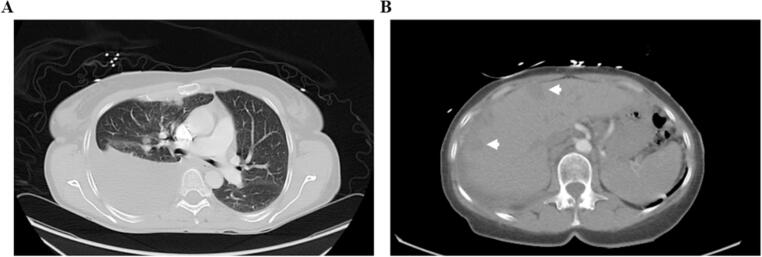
CT imaging of malignancy from March 2019 showing disease progression. (A) Section of lung parenchyma with large right-sided pleural effusion and calcified metastatic nodules. (B) Section of liver with likely hepatic metastases, indicated by arrows.
